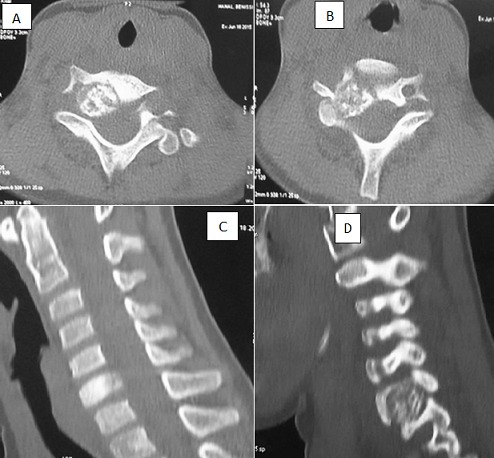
TDM en reconstruction axiale (A-B) et sagittale (C-D) en fenêtre osseuse de la même patiente de 7 ans objective une formation arrondie, bien limité siège d’ostéolyse et d’ostéocondensation du corps de C7 latéralisé à droite rétrécissant le trou de conjugaison de C7-D1 et le canal médullaire en regard arrive au contact du pédicule droit non vue à la radiographie: ostéoblastome
